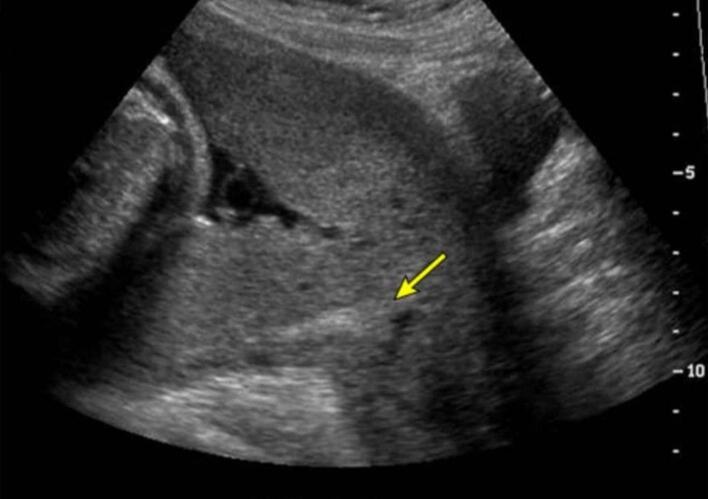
An abdominal ultrasound findings were as follows: several blood lakes within the placenta and a placenta previa totalis, which invaded the uterine wall.
